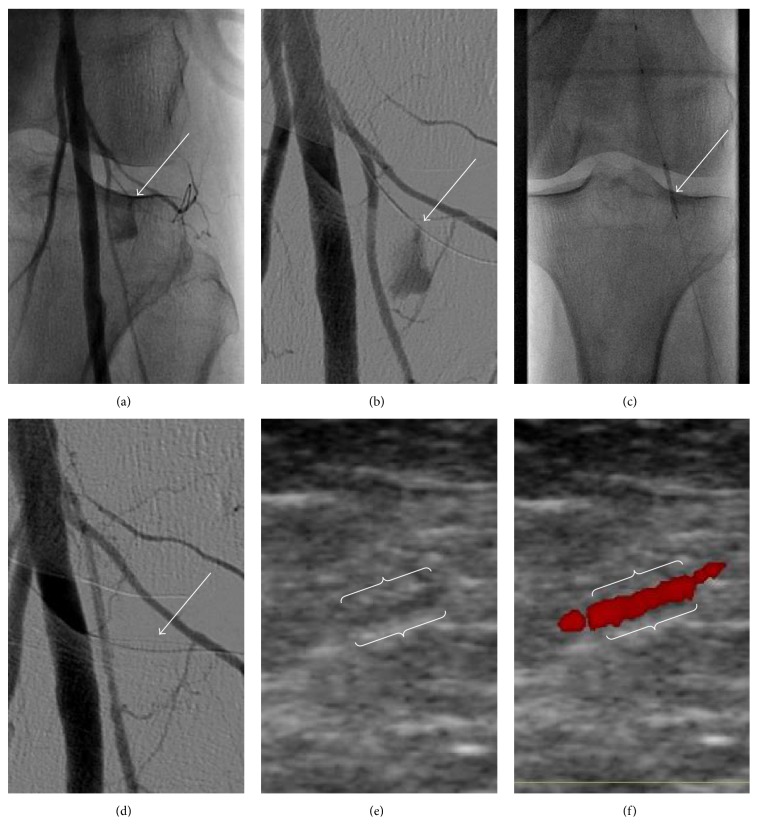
Perforation of a small side branch of the popliteal artery presumably by the 0.035′′ Terumo guide wire (white arrow in (a)). A 0.014′′ wire was subsequently inserted into this side branch (b), and bleeding was stopped after placement of a coronary Direct-Stent stent graft (c and d). Using colour doppler ultrasound the stent graft (white brackets) could be visualized one day after implantation, exhibiting normal blood flow (e and f).
